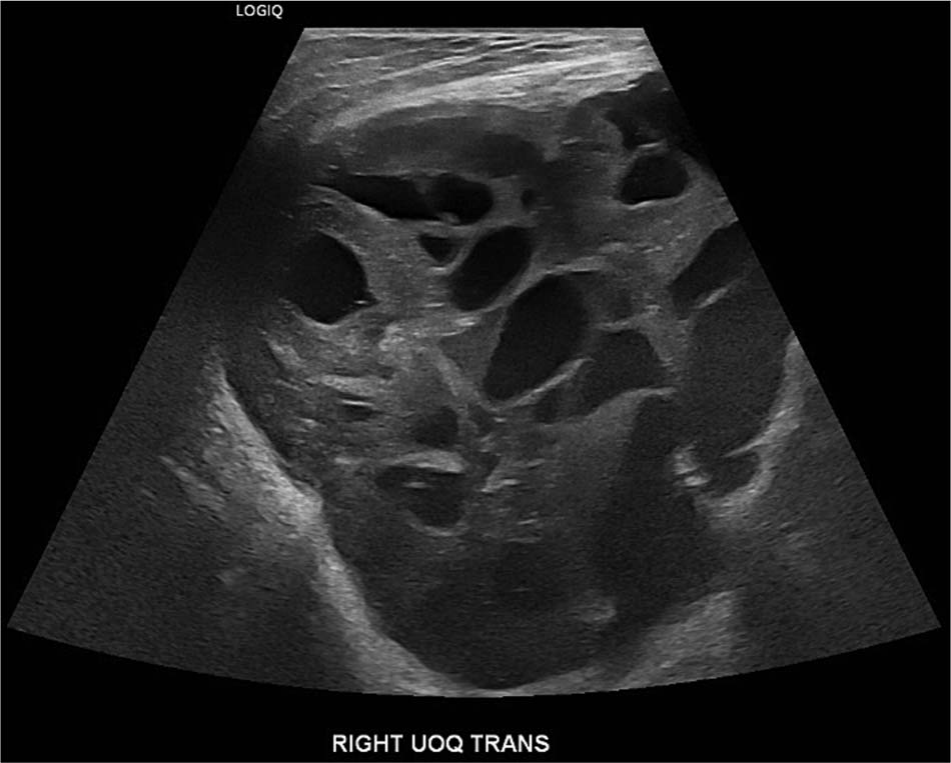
An ultrasound at that time revealed a large complex mass measuring 19 × 17 × 23 cm, with multiple solid and cystic components and no increased vascularity, consistent with BI-RADS 4.
